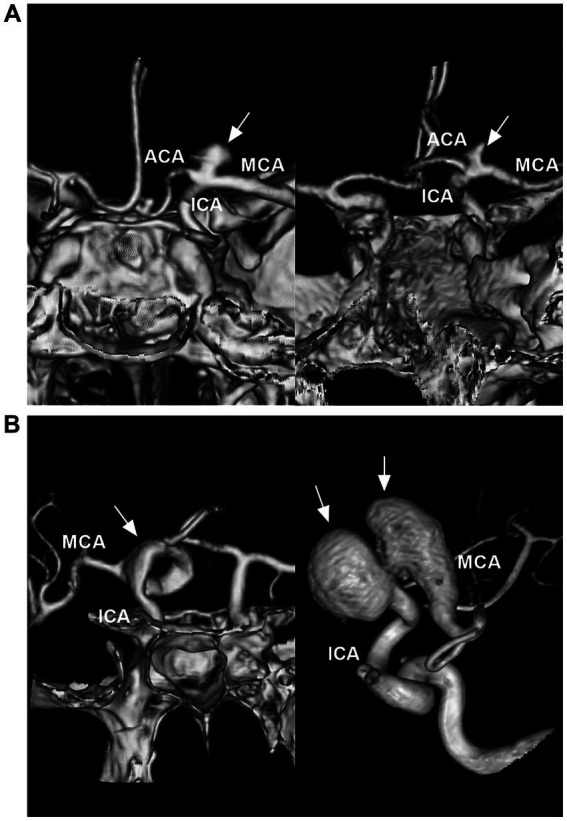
Classifications of ICA bifurcation region aneurysms. (A) Left panel: CTA showing a symmetric type of saccular aneurysm (arrow) that is on the highest point of the ICA. Right panel: CTA showing a M1 predominant type of saccular aneurysm (arrow). (B) Left panel: CTA showing a serpentine aneurysm (arrow) involving the ICA terminus. Right panel: Three-dimensional DSA showing a tandem of dissecting aneurysms (arrows) involving the ICA terminus and MCA origin. ACA, anterior cerebral artery; CTA, computed tomography angiography; DSA, digital subtraction angiography; ICA, internal carotid artery; MCA, middle cerebral artery.
